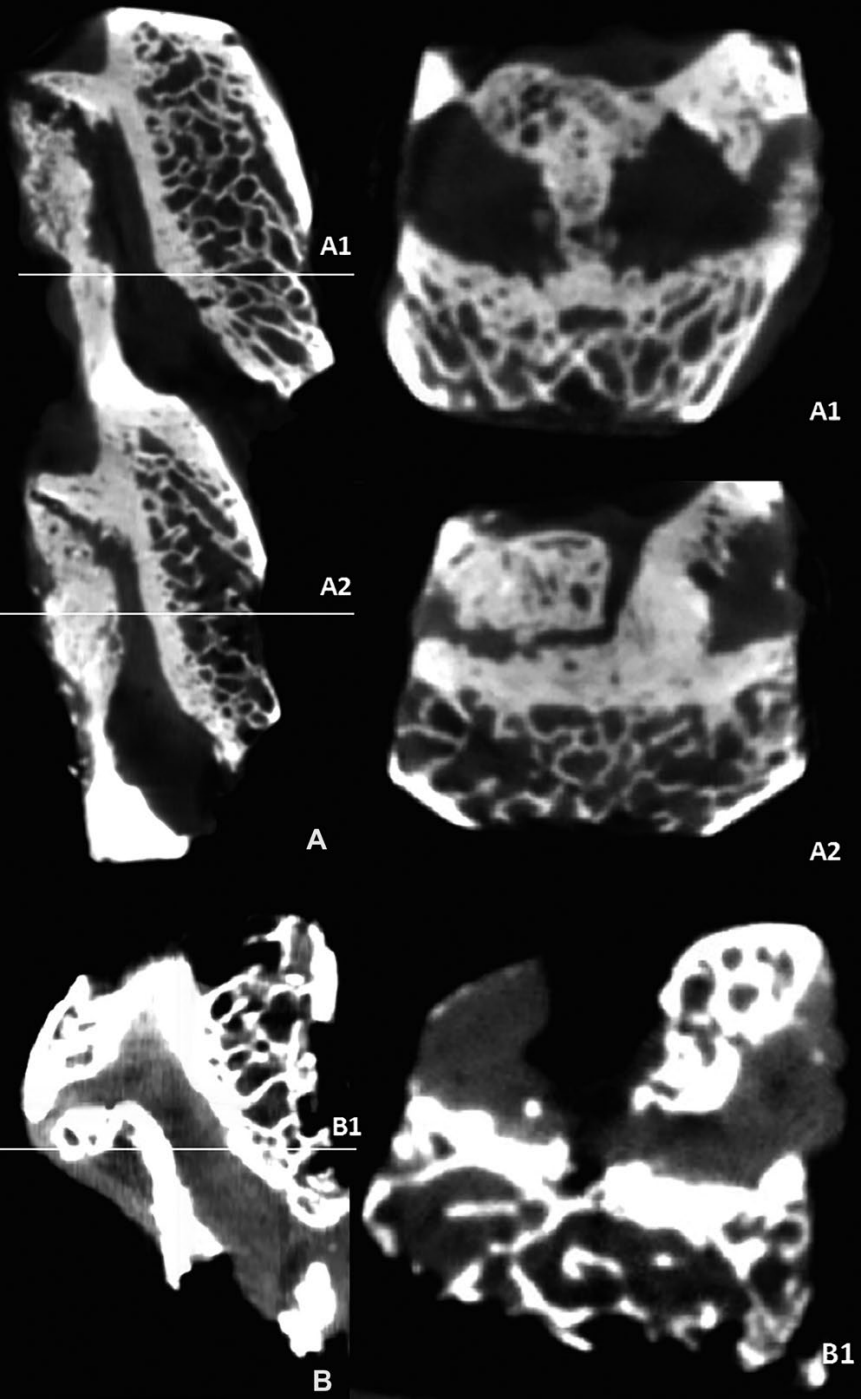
Micro‐CT images of TOLF ossification. A, Micro‐CT images of multiple‐level TOLF and its horizontal section A1and A2, (B) micro‐CT images of single‐level TOLF and its horizontal section B1. CT, computed tomography; TOLF, thoracic ossification of the ligamentum flavum
